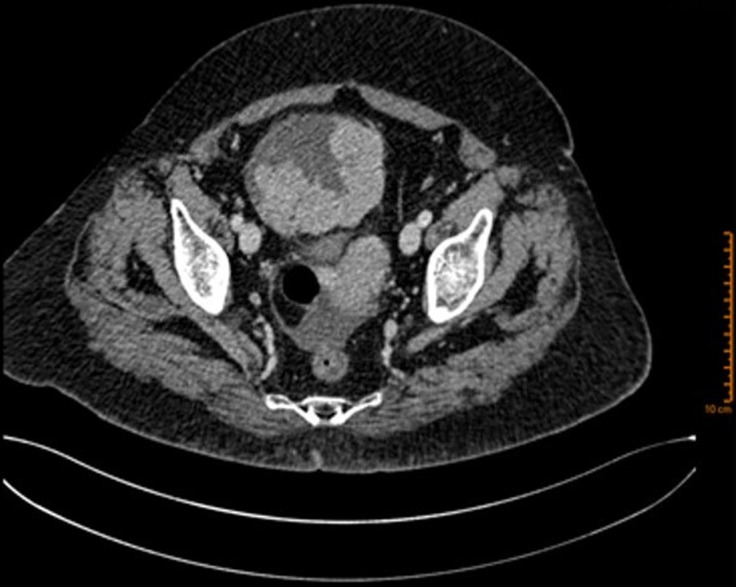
abdominal-pelvic CT scan showing an oval, well-limited right ovarian mass, with a prevailing cystic component and a solid component enhanced after injection of contrast agent
